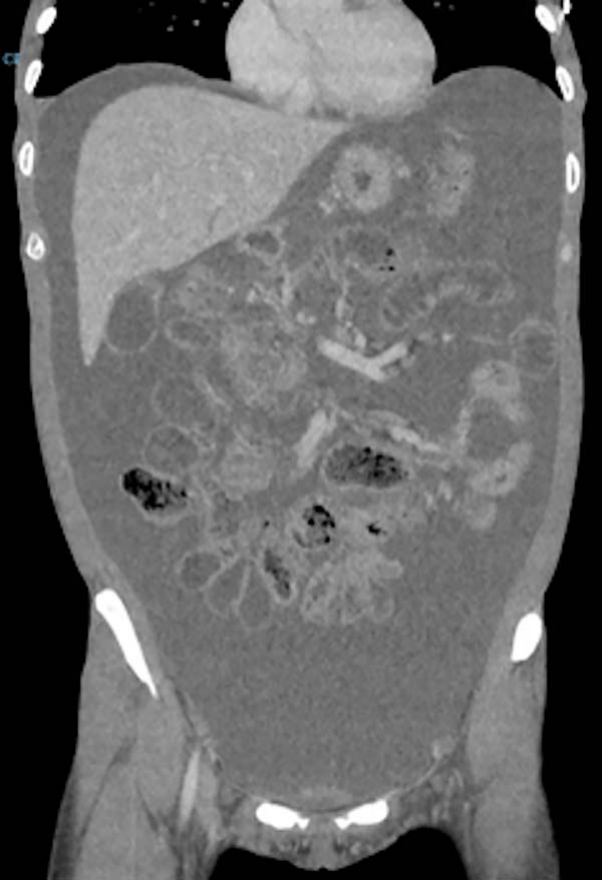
Initial CT scan performed on admission.
